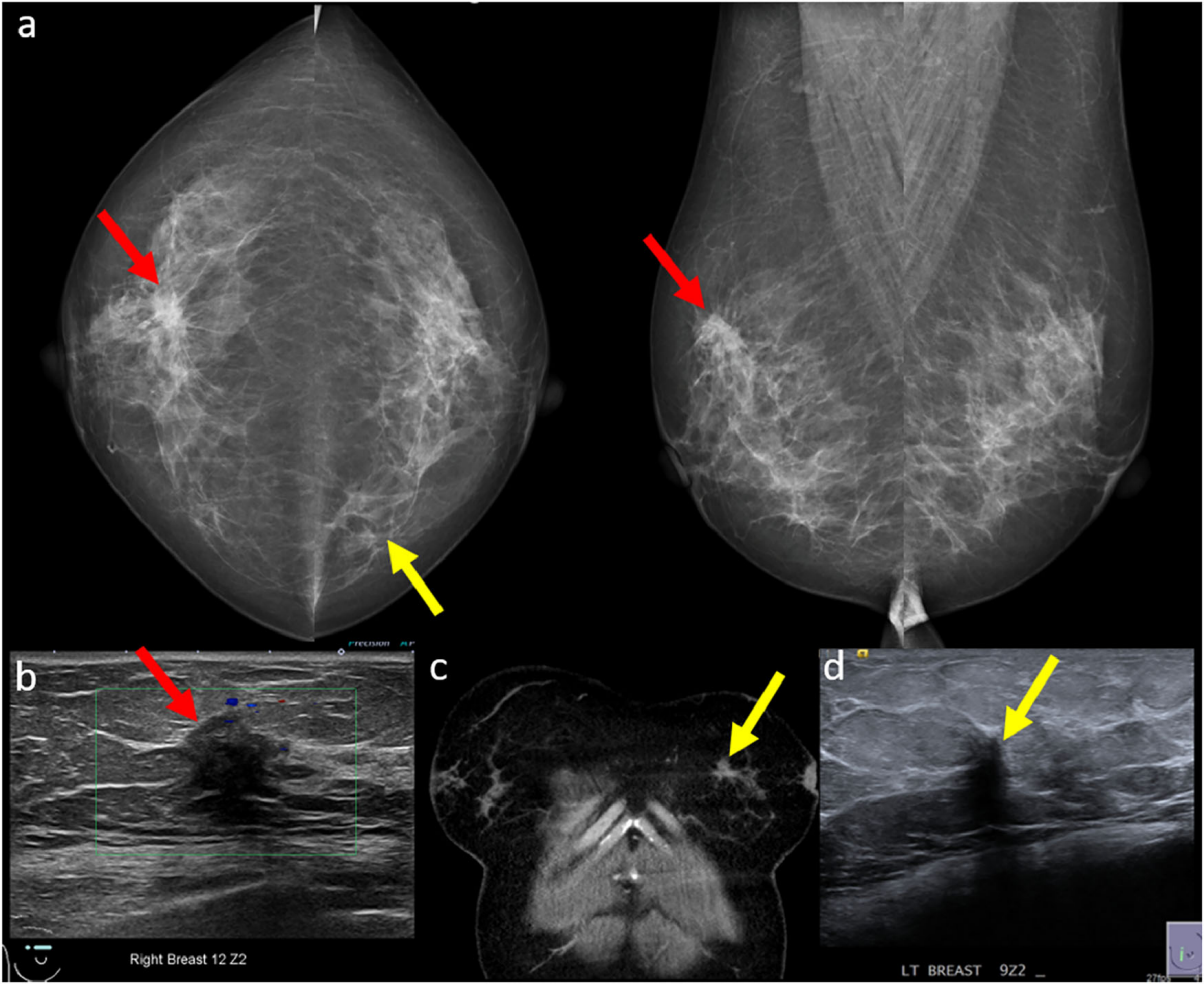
a Mammogram of a 48-year-old woman with a palpable right breast lump showed a spiculated high-density mass in the upper central right breast (red arrows). b Corresponding ultrasound showed an irregular hypoechoic mass with angular margins (red arrow) at 12 o’clock. Ultrasound-guided core biopsy revealed invasive ductal carcinoma. c Staging CT (coronal image) showed a spiculated mass in the contralateral (left) breast (yellow arrow). Retrospective review of the screening mammogram identified a previously overlooked asymmetry in the medial left breast (yellow arrow). d CT-directed breast ultrasound of the left breast demonstrated an irregular hypoechoic mass with indistinct margins and posterior shadowing at 9 o’clock (yellow arrow), corresponding to the CT finding. Ultrasound-guided core biopsy revealed invasive tubular carcinoma
